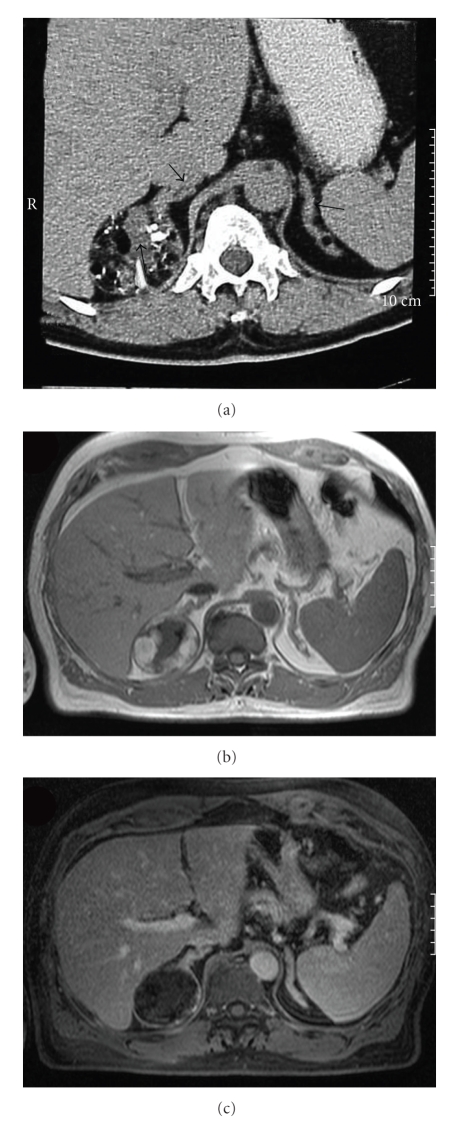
Computed tomography of the adrenals in Patient 1. (a) Arrows show a 5.7 centimetre large, expansive process in the right adrenal with a heterogeneous stroma, fat, calcifications, and in addition, pronounced bilateral adrenal hyperplasia. Magnetic resonance images of the adrenal tumor, T1 Weighted Imaging, with hyperintense fat (b) showing signal loss in fat suppressed image (c).
